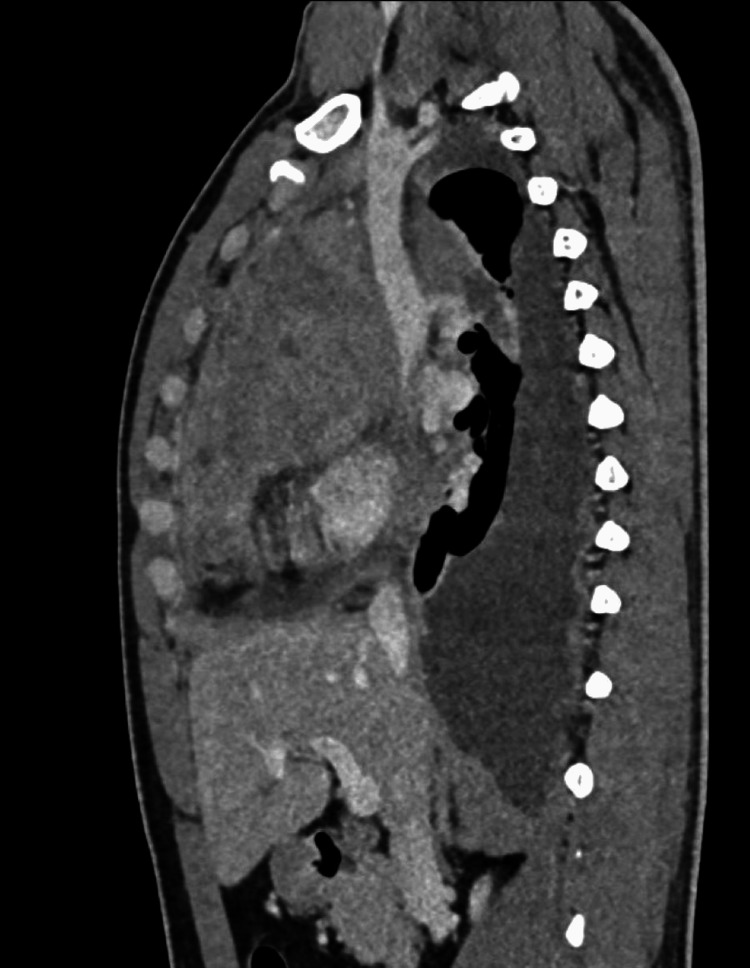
Contrast-enhanced computed tomography scan: sagittal section of mediastinal window showing an anterior mediastinal mass with right side pleural effusion.
